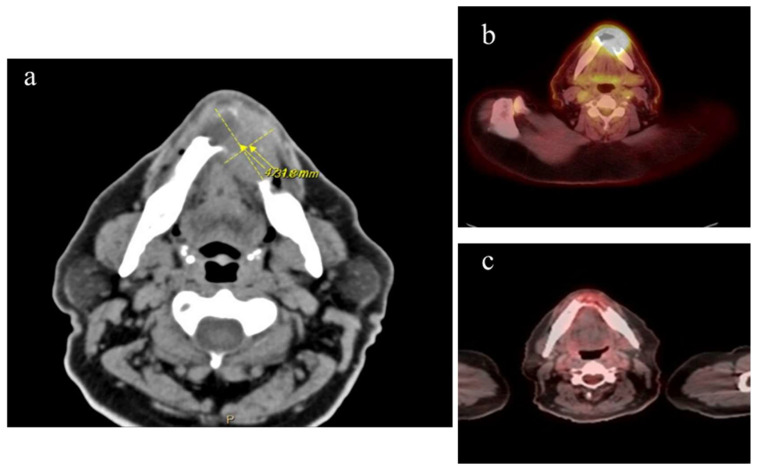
A 64-year-old M with DLBCL stage IE. (a) CT imaging of mandible with mass and associated bone destruction at the time the lymphoma diagnosis was made, 9 months after initial presentation with loose teeth and shortly after completing a 6-week course of oral antibiotics. (b) PET scan taken during initial lymphoma staging, with uptake in mandibular area SUV 18.8. (c) PET scan after completion of 3 cycles of chemotherapy, showing complete remission.
